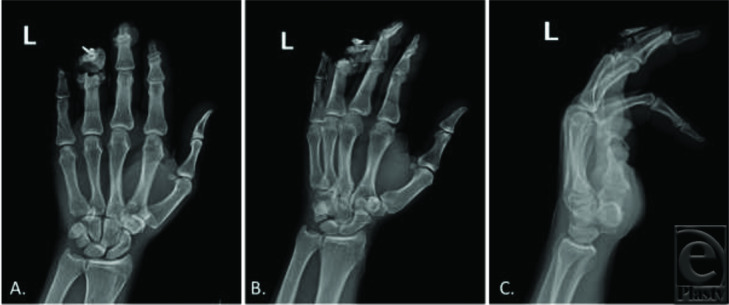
Three-view preoperative radiographs: (*a*) Posteroanterior, (*b*) oblique, and (*c*) lateral, demonstrating a left-hand small finger amputation distal to the level of the DIP joint, a near amputation and comminuted ring finger middle phalanx head fracture, and a long finger fracture-dislocation involving the DIP joint and distal phalanx. DIP indicates distal interphalangeal.
